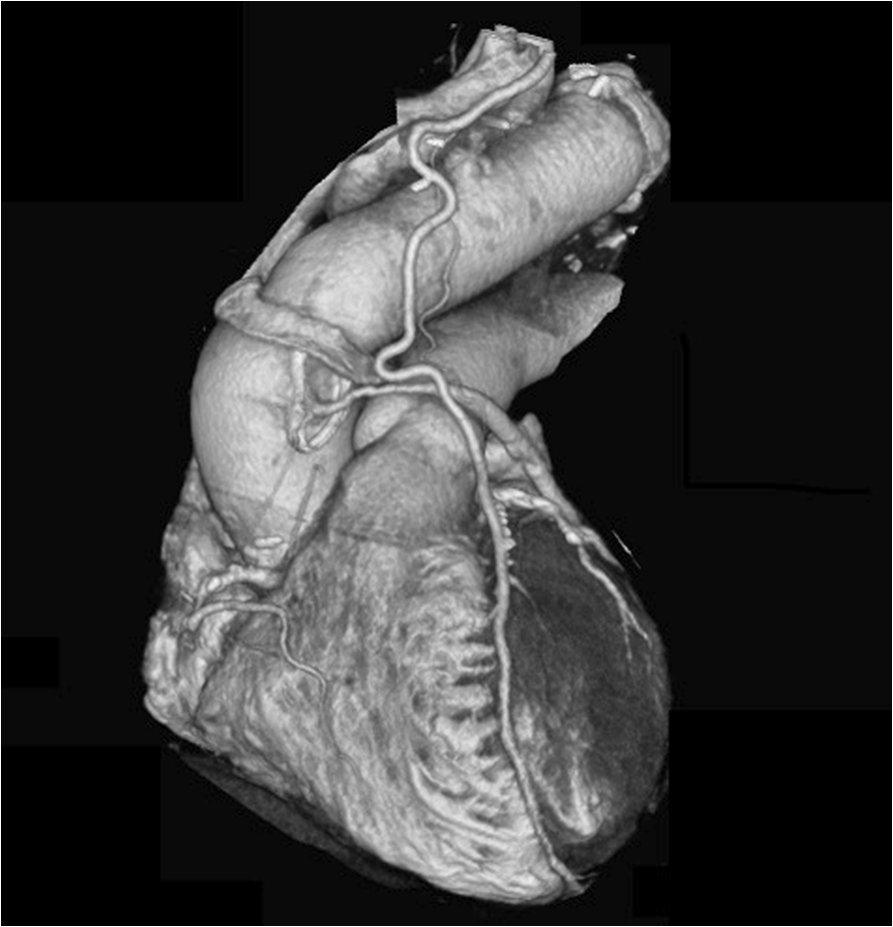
Postoperative CT scan. Postoperative CT scan of woven Dacron graft that replaced aortic arch.
